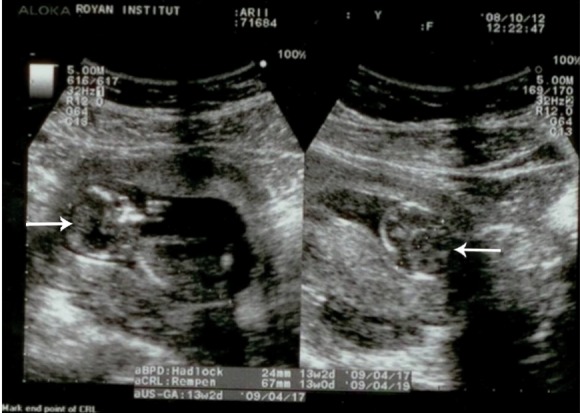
This is a transabdominal sonography in a 26-year
